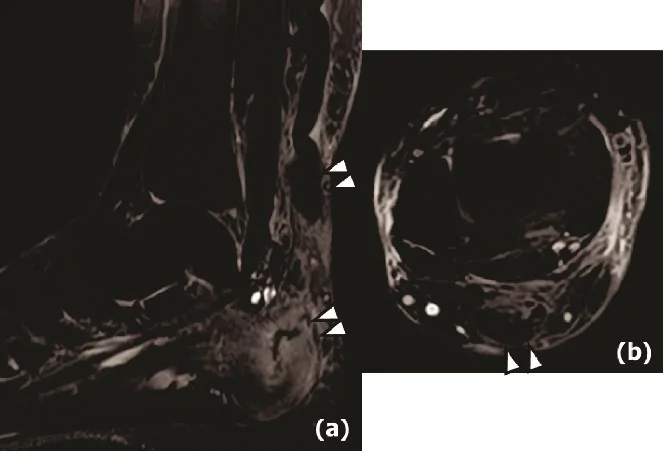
Preoperative MRI (T2 fat-suppressed, sagittal view) showing bone marrow edema of the calcaneus. (a) Sagittal image, (b) axial image.
